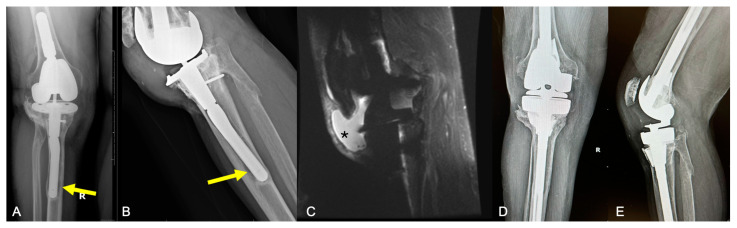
A 67-year-old male with a remote history of complex endoprosthetic reconstruction after resection of giant cell tumor of bone of the proximal tibia, now presenting 40 years after the initial reconstruction with knee pain and inability to extend the knee after kneeling. (A) AP and (B) lateral radiographs show a hinged knee arthroplasty with patella alta and prepatellar soft tissue swelling suggestive of patellar tendon tear. Also present is a concomitant aseptic loosening of the tibial component (yellow arrows) with posterior stem migration in relation to the initial soft tissue failure. (C) Sagittal fat-suppressed T2-weighted MRI confirms a full-thickness patellar tendon tear, with a large infrapatellar defect (*) that allowed wear-induced synovitis and joint effusion to decompress into the pretibial soft tissues, constituting a type 1A failure. Other surrounding ligaments show poor scar remodeling. The patient underwent revision total knee arthroplasty with extensor mechanism reconstruction using the Mayo Clinic Marlex Mesh [21], with (D) AP and (E) lateral radiographs taken 4 months post-operatively demonstrating secure femoral and tibial components.
